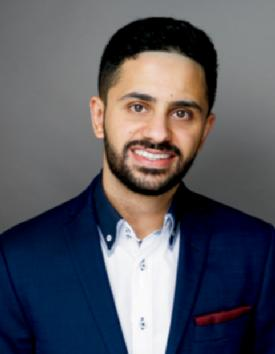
Dr. El-Hisnawi obtained his medical degree at the TU Dresden (Germany). He is a cardiology resident at the Vivantes Neukoelln Hospital in Berlin. Areas of interest include electrophysiology, heart failure, cardiotoxicity, and echocardiography.
